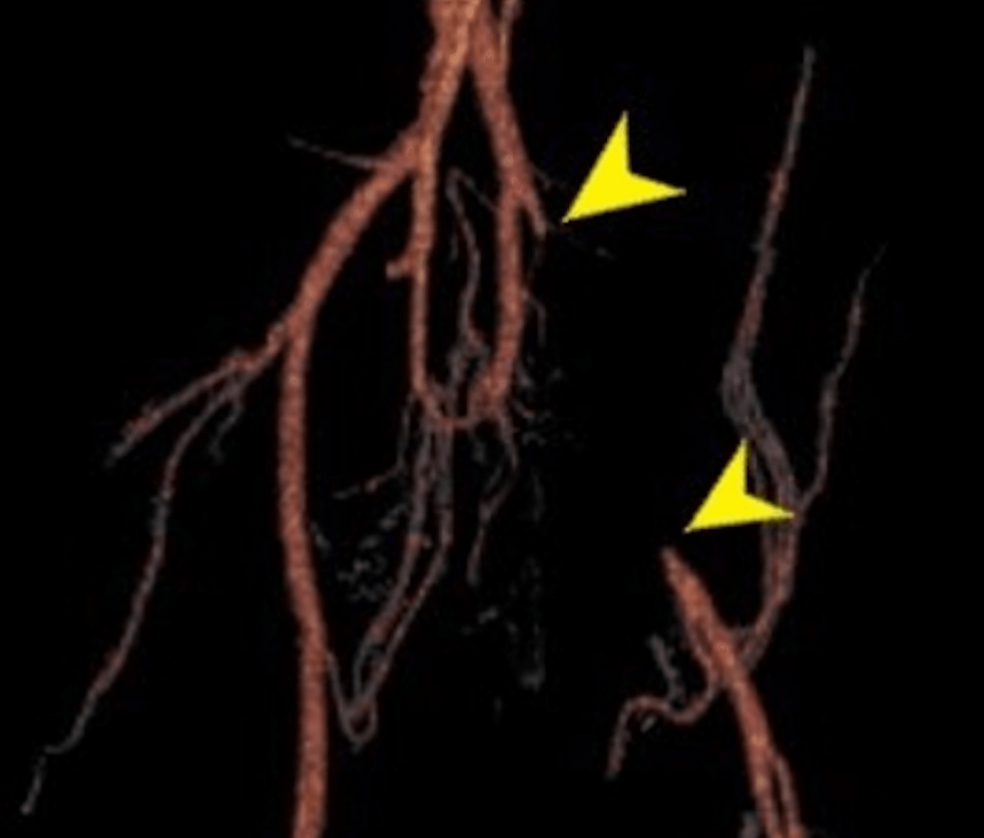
CTA bilateral lower extremities with 3D reconstruction of case 2 CTA of the bilateral lower extremities with 3D reconstruction displaying the extent of atresia stemming from the proximal left external iliac artery.
